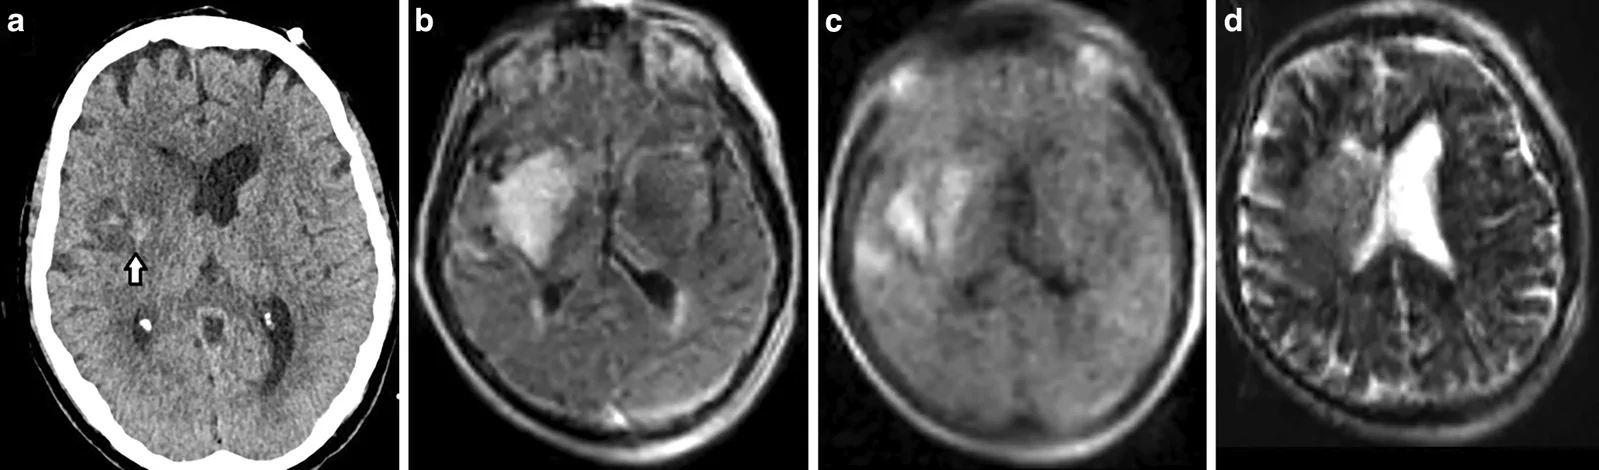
Hemorrhagic transformation (HBC 1b) was detected on CT (arrows in a) but was not visible on ULF-pMRI (b–d) in a right basal ganglia infarction following occlusion and subsequent recanalization of the right middle cerebral artery. CT and MRI were performed one day later in a 65-year-old patient
